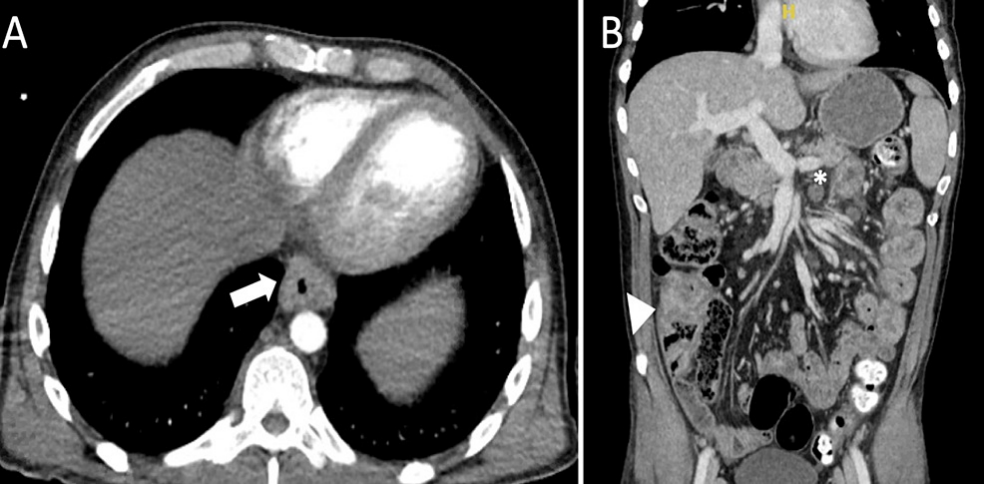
Computed aomography angiography abdomen and pelvis on initial presentation. Computed tomography angiography with (A) mural thickening in the mid and distal esophagus (arrow) and (B) new mesenteric adenopathy (asterisk) and localized wall thickening of ascending colon (arrowhead).
